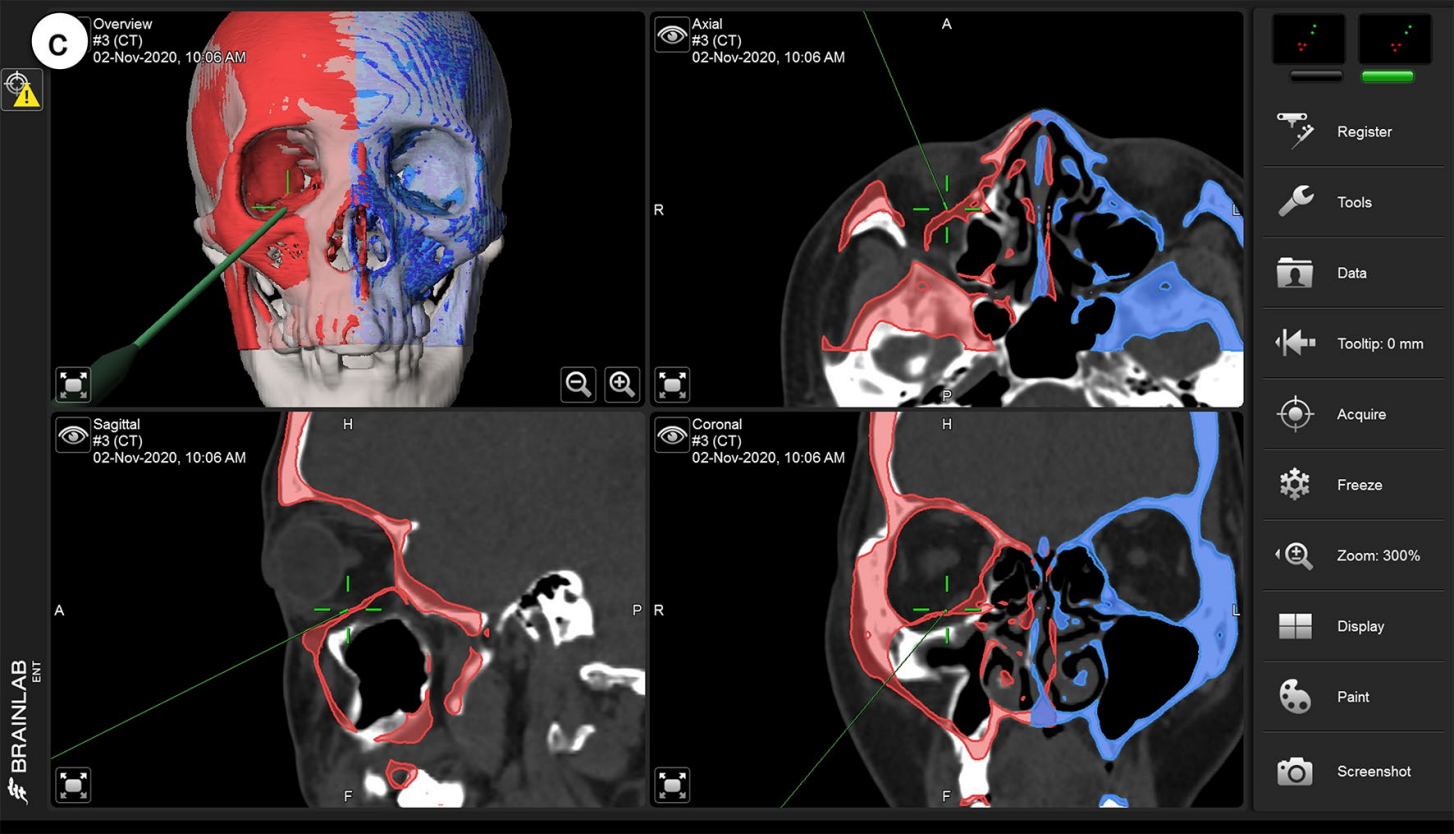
(a) Intraoperative real-time navigation for confirmation of zygomatic bone position at the zygomatic prominence. The semiautomated segmentation objects of the digital template were created on the unaffected side (blue) and superimposed onto the preoperative CT images, then they were mirrored to the affected side (red), resulting in an ideal presurgical plan with symmetrical positioning of the bones. The real-time position of the reduced zygoma prominence was confirmed by the laser pointer in the axial, coronal, and sagittal views. CT, computed tomography. (b) Intraoperative real-time navigation for confirmation of zygomatic bone position near the zygomaticofrontal junction. The real-time position of the reduced zygoma near the zygomaticofrontal junction was confirmed by the laser pointer in the axial, coronal, and sagittal views. (c) Intraoperative real-time navigation for confirmation of reconstructed implant position on the orbital floor. The real-time position of the reconstructed implant is shown, as verified on the orbital floor by the laser pointer in the axial, coronal, and sagittal views.
